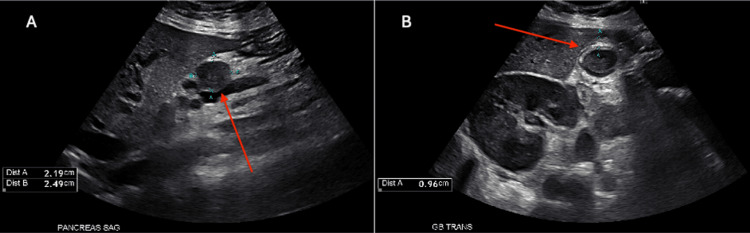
Abdominal ultrasound demonstrating pancreatic head lesion and biliary abnormalities (A) Sagittal view of the pancreas showing a complex echogenic lesion in the pancreatic head measuring approximately 2.2 × 2.5 × 2.3 cm (red arrow). The surrounding liver parenchyma demonstrates diffusely increased echogenicity, consistent with fatty infiltration. (B) Transverse view of the gallbladder demonstrating a dilated common bile duct measuring approximately 0.96 cm with associated gallbladder wall thickening and biliary sludge (red arrow), raising concern for biliary obstruction in the setting of pancreatic head involvement.
